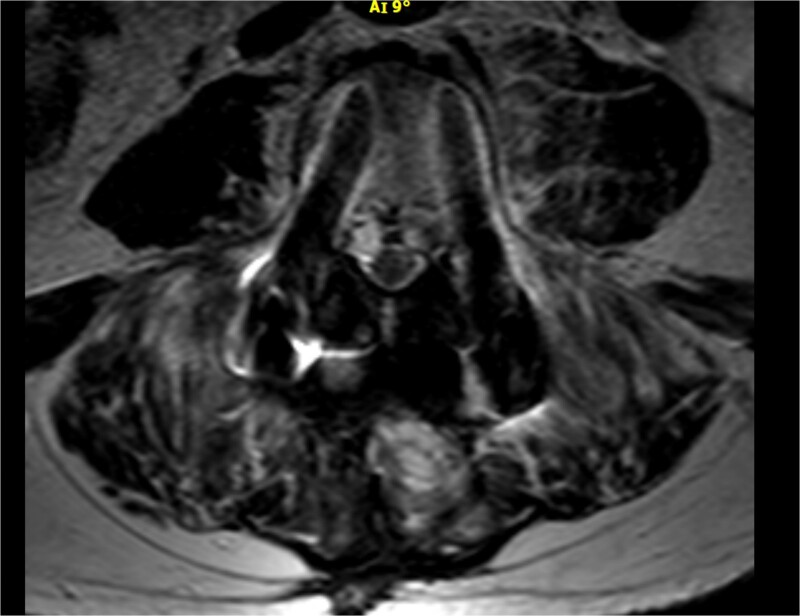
Axial view of the MRI scan demonstrating pathological fluid collection around the pedicle screws.
